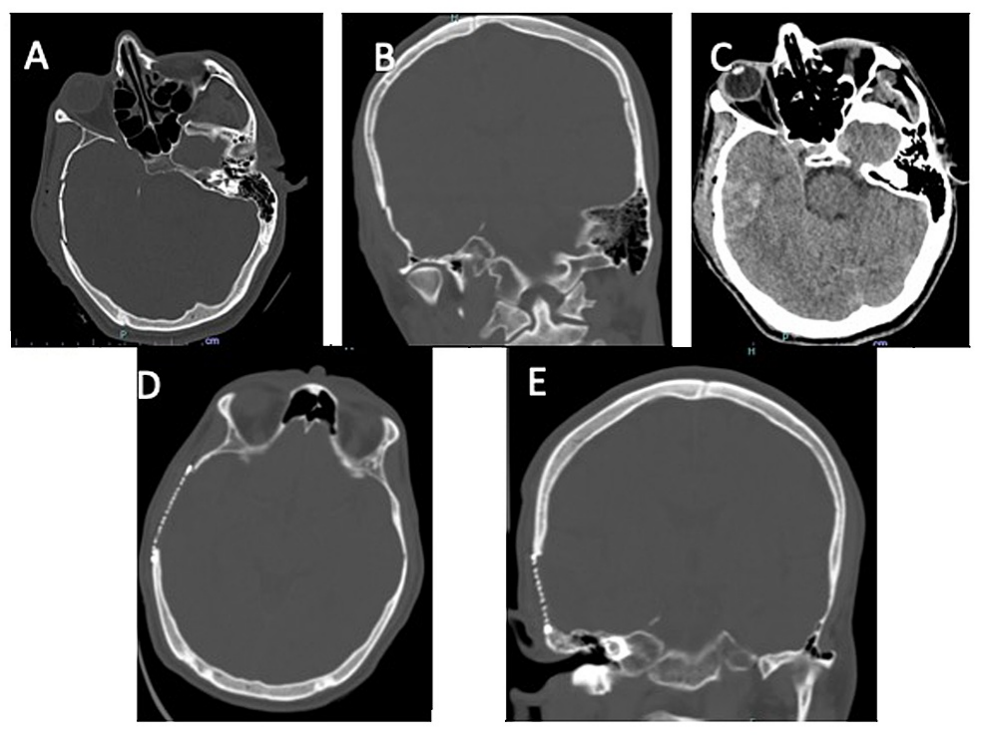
Neuroimaging findings of Case #3 A-B: Axial and coronal views on CT of the head revealing acute comminuted depressed right temporal bone fracture, C: Right temporal lobe contusion underlying the right temporal bone fracture, D-E: Postoperative CT of the head revealing titanium mesh cranioplasty following elevation and repair of the depressed temporal bone skull fracture
